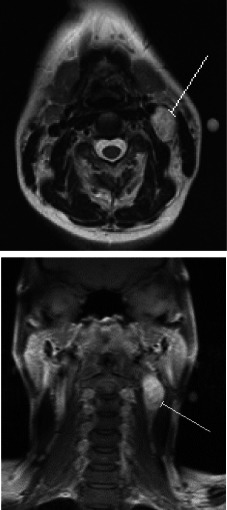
Axial and coronal views of contrast enhanced MRI (labels to ancient schwannoma).
